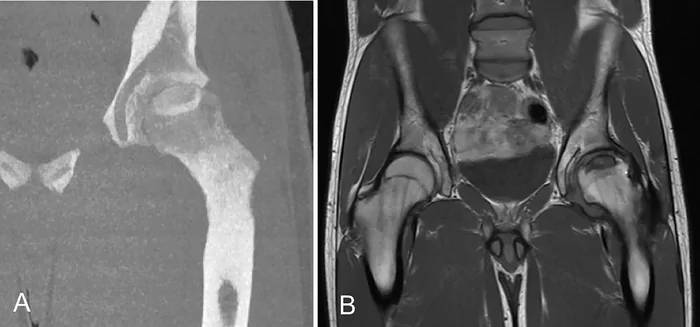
(A) Computed tomography scan at 24 months postoperatively shows maintained contour and alignment, demonstrated sclerosis, and no collapse. (B) Bilateral hip T1 magnetic resonance imaging shows a maintained bone and articular cartilage structure with poor vascularity of the graft.
